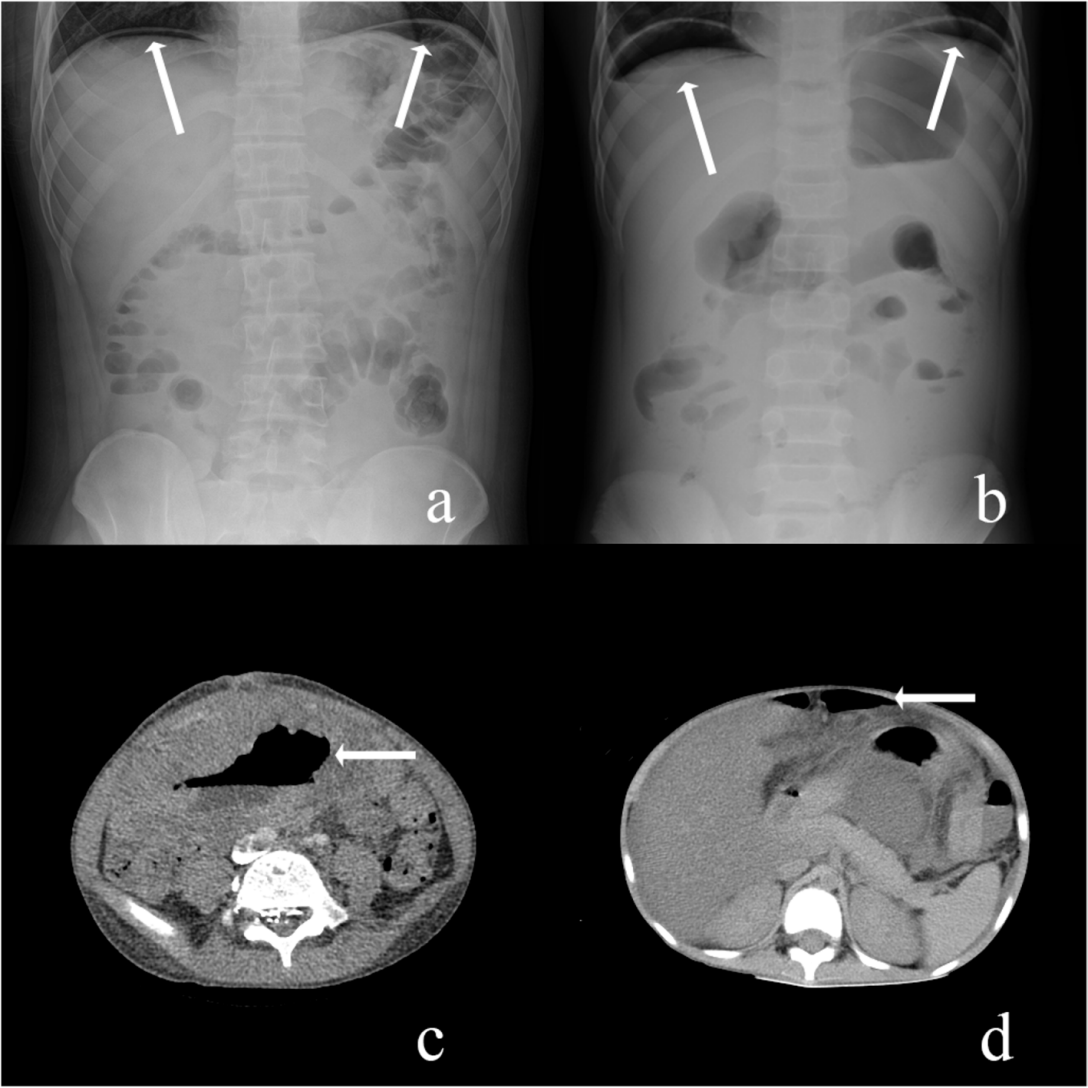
Perforation confirmed by radiology imaging (white arrow: free gas). a a little free gas with decreasing under the diaphragm by abdominal X-ray. b a lot of free gas without decreasing under the diaphragm by abdominal X-ray. c localized intra-abdominal free gas around the colon by abdominal CT. d diffuse intra-abdominal free gas in front of the liver by abdominal CT
